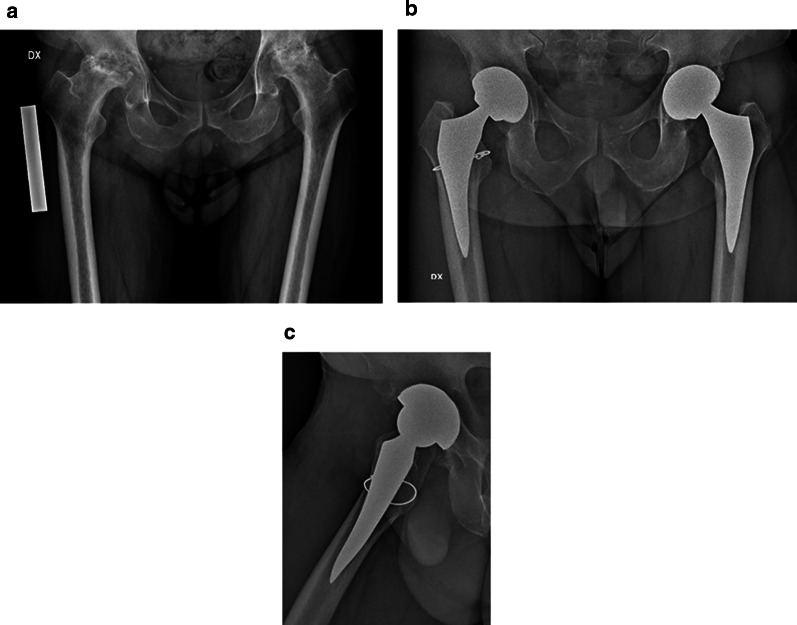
Bilateral avascular necrosis of femoral head in a 46-year-old patient (a). Five years radiographic follow-up: anteroposterior view (b) and axial view of right (c) and left (d) hips. Undisplaced fracture of calcar region treated with preventive cerclage on right hip
